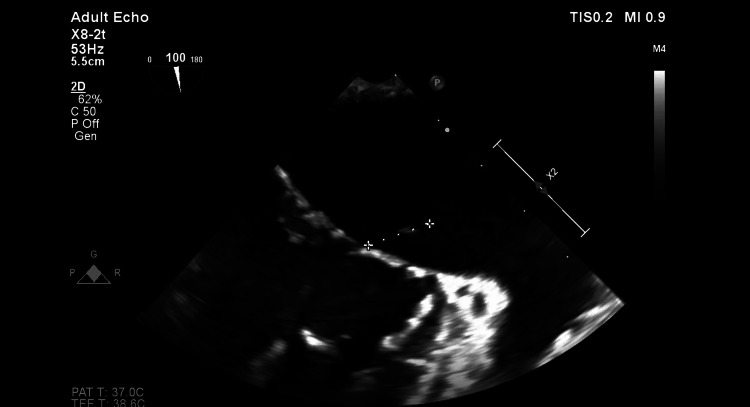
Image from echocardiogram, with evidence of endocarditis of the mitral valve.
